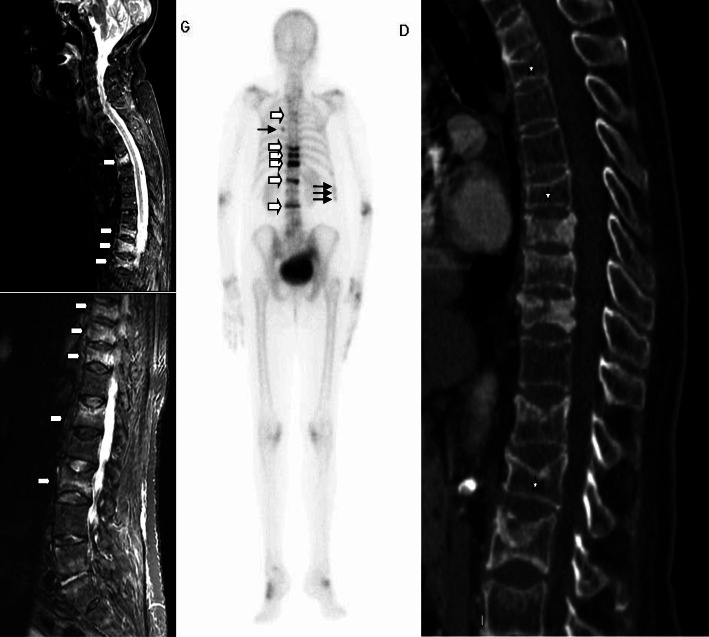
Bone scintigraphy, computed tomography scan, and magnetic resonance imaging in June 2014. Magnetic resonance imaging and bone scintigraphy confirmed that there were six recent vertebral fractures (fourth thoracic vertebra, ninth thoracic vertebra, tenth thoracic vertebra, 11th thoracic vertebra, first lumbar vertebra, and third lumbar vertebra): *small white arrows*. There were some costal fractures on bone scintigraphy: sixth posterior on the right side and laterally tenth, 11th, and 12th on the left side (*black arrows*). A computed tomography scan also showed old vertebral fractures (fifth thoracic vertebra, eighth thoracic vertebra, and second lumbar vertebra): *white stars*
